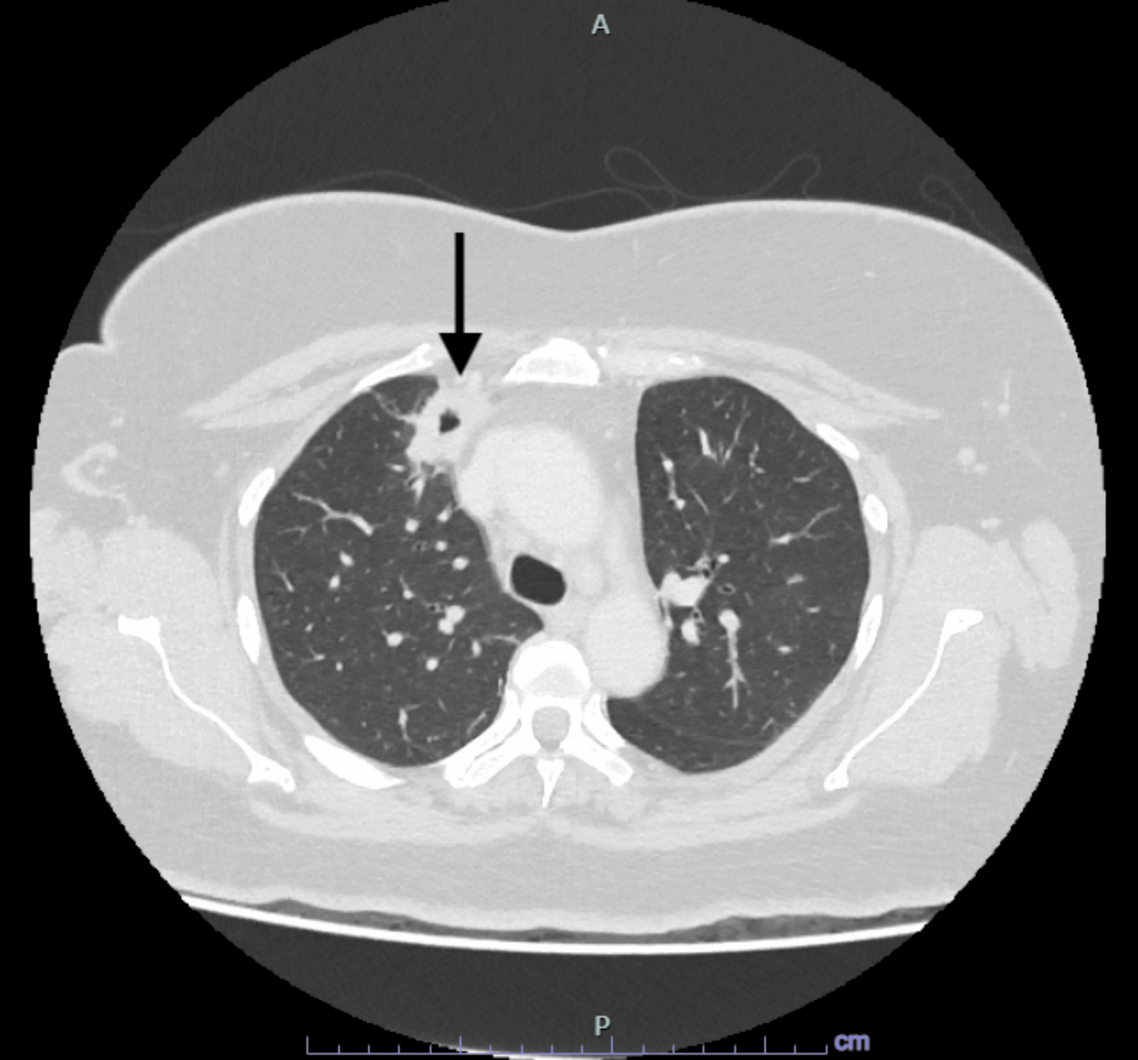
Interval CT Scan of thorax after four weeks of effective antibiotics showing significant improvement with a smaller nodule at the right upper lobe, measuring 2.8 x 1.7 x 3 cm. A: anterior, P: posterior
